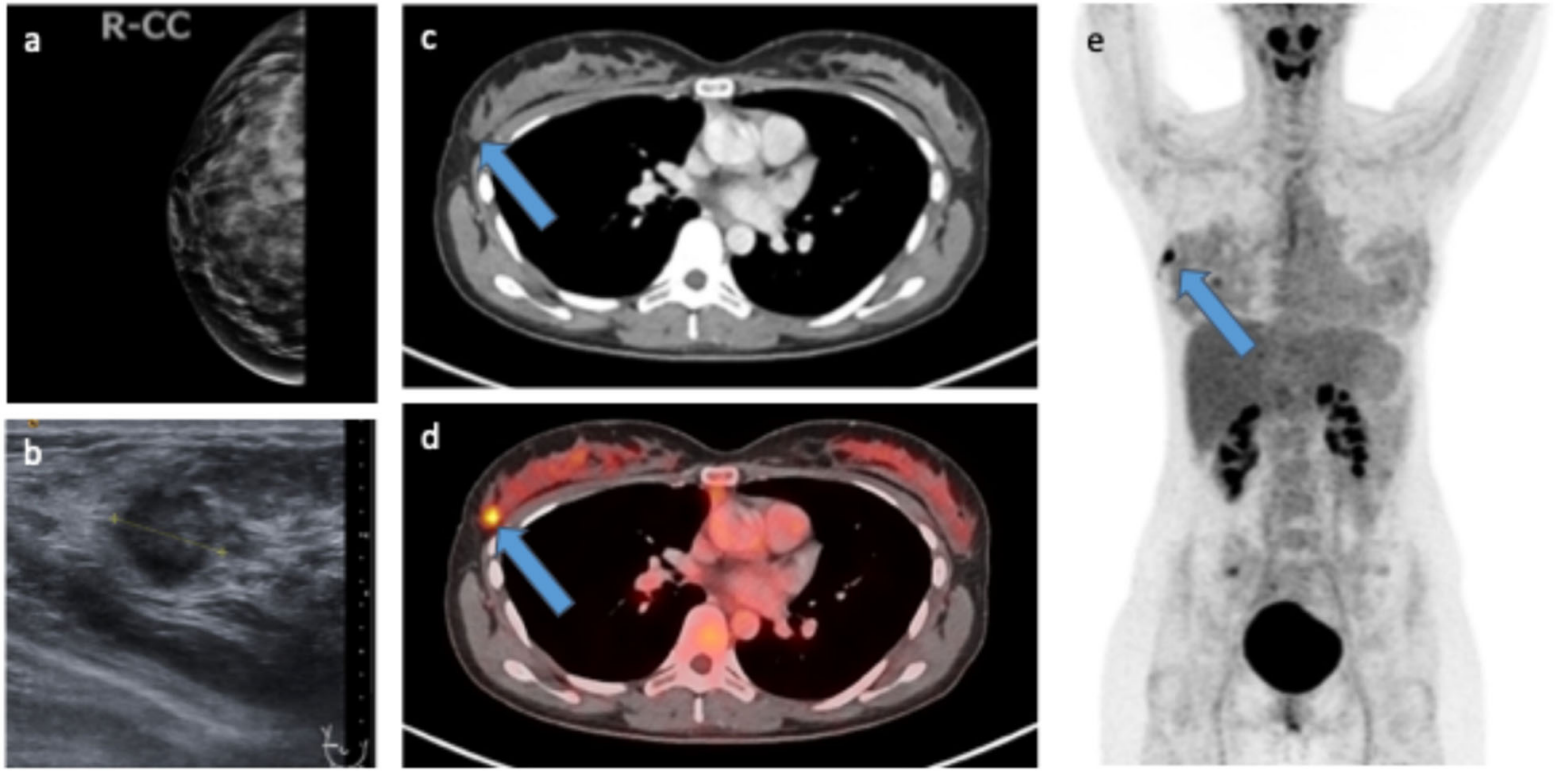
Due to the dense breast tissue (a), no suspicious lesions were detected on mammography. Ultrasonography of the right breast measured a 1.5 cm lesion (b). Given the patient’s young age (aged 30) and clinical suspicion of metastases, FDG-PET/CT was performed (c–e). FDG-avid lesion in the right lower outer quadrant (d) was not clearly identified on CT (c). The right axillary lymph nodes were not enlarged and showed no suspicious FDG uptake. The maximum intensity projection also shows a singular lesion in the right breast (e). No additional lesions suspicious for metastases were detected
